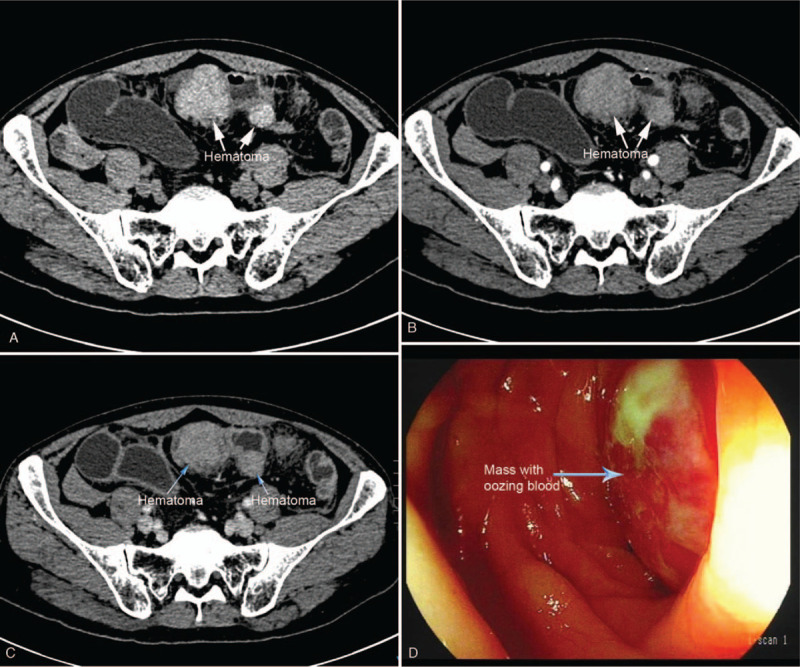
(A–C) Abdominal CT revealed multiple hyperdense masses in the sigmoid colon without enhancement after contrast administration. (D) Colonoscopy showed a submucosal mass with intact mucosa and oozing blood on its surface. CT = computed tomography.
